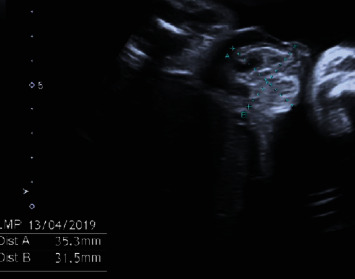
In the ultrasound examination performed at the 32nd week of gestation, the allantoic cyst was no longer visible and replaced by an omphalocele of 35 × 31 millimeters, secondary to the cyst's rupture.
